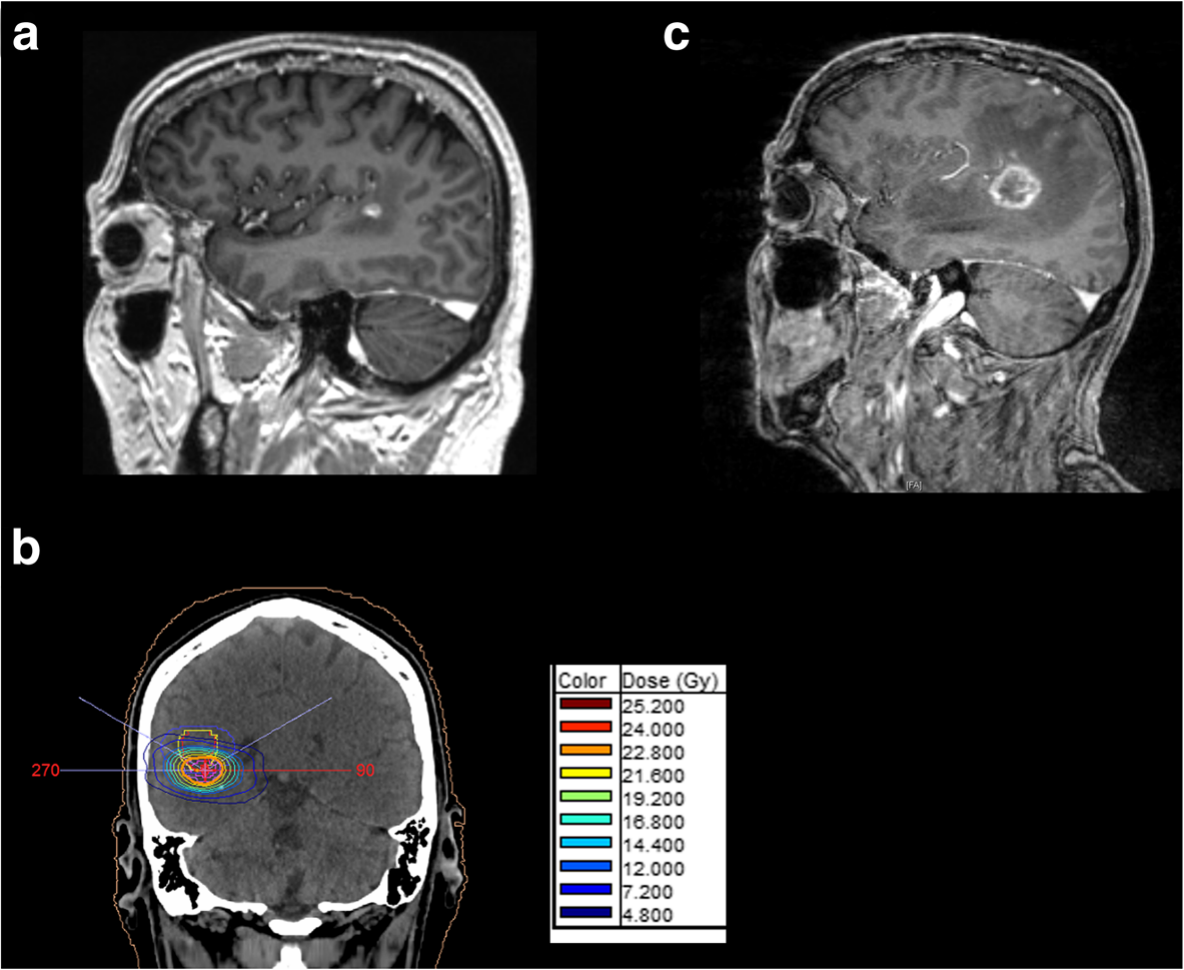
Magnetic resonance imaging of intracranial lesions. a Parieto-temporal lesion that was irradiated. T1 weighted MRI after application of Gadolinium-containing contras material. b Presentation of the radiation field that was applied to the lesion. c Progression of irradiated lesion after irradiation and before resection of the lesion
